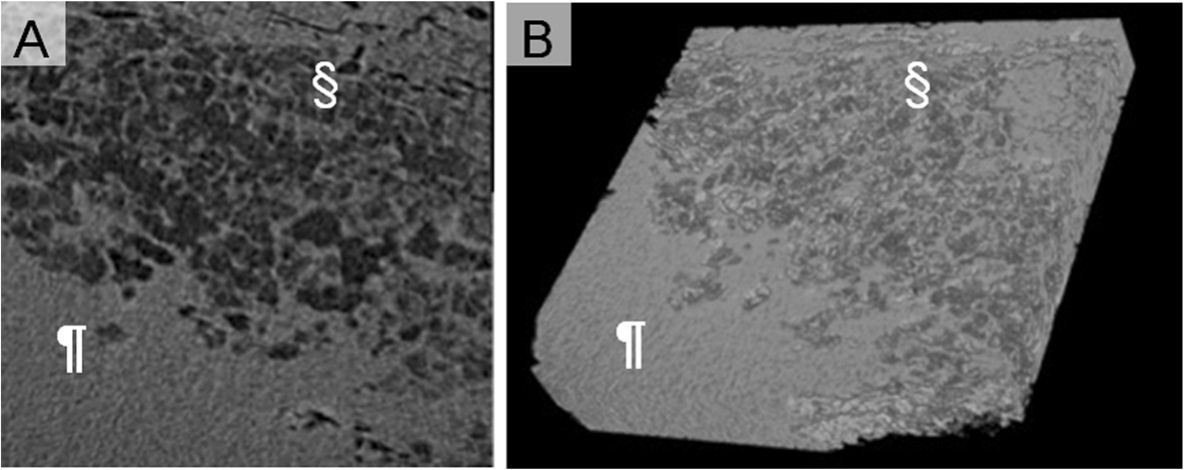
Radiological examinations in patient with invasive pulmonary aspergillosis presenting discrete nodule. (A) Micro computed tomography image of the lung tissue with invasive pulmonary aspergillosis. The pilcrow (¶) and the section signs (§) are placed on the areas of monotonous high density and peripheral reticular structure, respectively. (B) 3D reconstruction images processed by applying 3D volume rendering software. Central monotonous high density and peripheral fine reticular pattern were observed.
